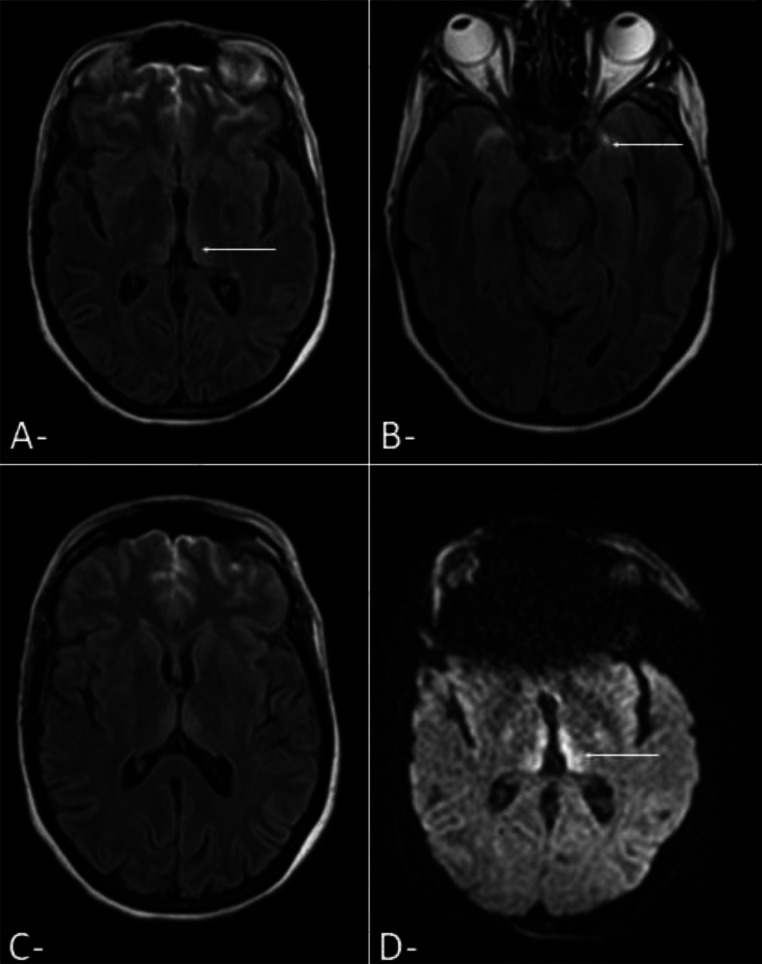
Emergency MRI images in axial cross-section T2 (A-C) and diffusion (D) revealing bilateral symmetrical cortical hypersignal in the dorso-medial thalami, peri-aqueductal regions, and mammillary bodies.
